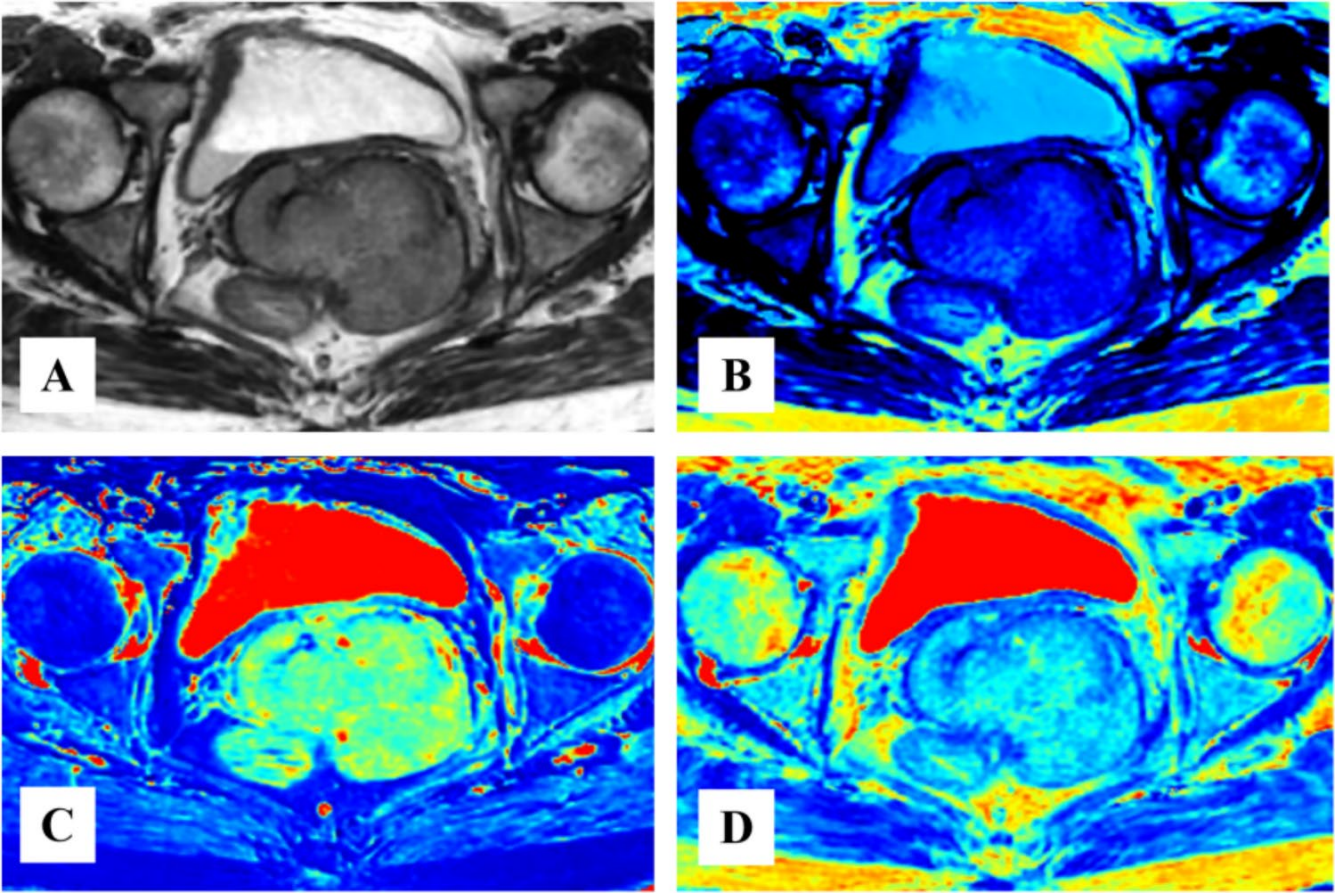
Representative synthetic magnetic resonance images (MAGiC) of a 38-year-old female with cervical cancer includes A synthetic T2WI (sy-T2WI), B T1 map, C T2 map, and D PD map, respectively
